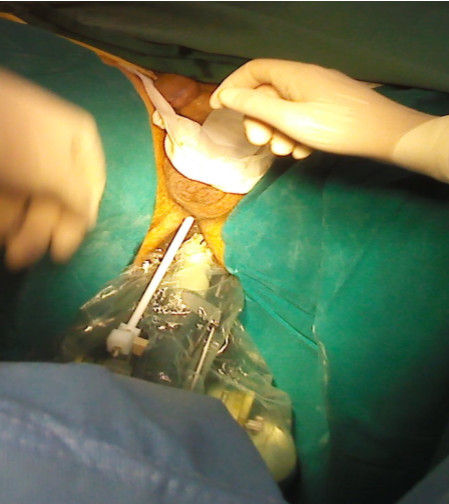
Placement of the introducer sheath under ultrasound guidance.
